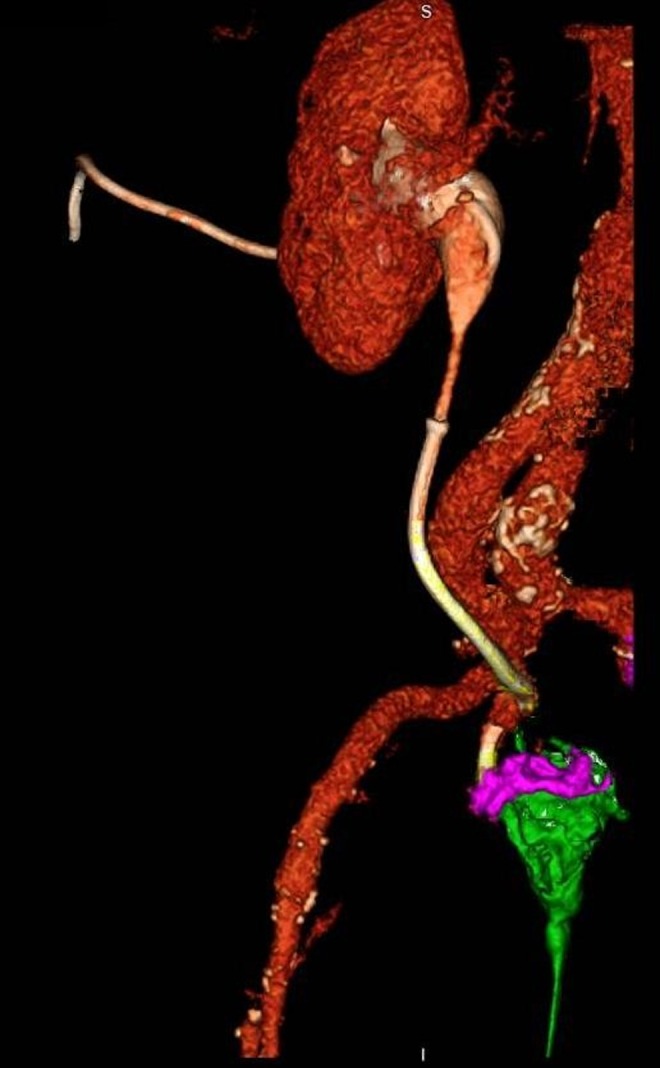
CT 3D reconstruction of right ureteroexternal iliac artery fistula and right ureteropresacral space fistula communicating to perineal sinus. *Green:* contrast in presacral bed draining through perineal sinus, *pink:* contrast in bladder, *yellow:* Memokath 051 stent.
